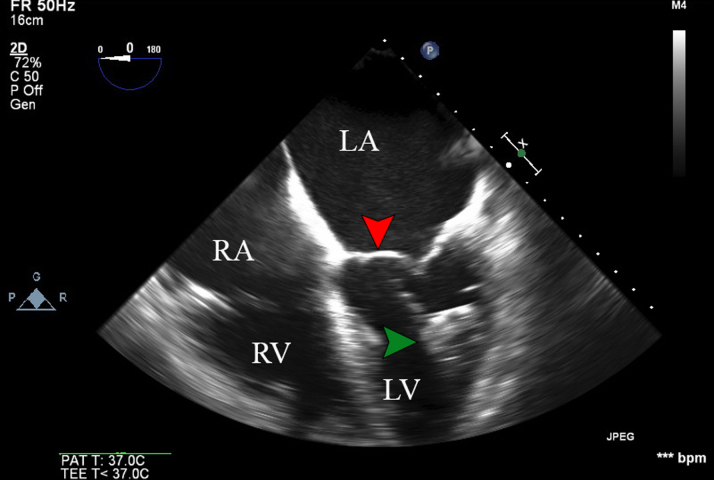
TEE Midesophageal 4-Chamber View Showing an Embolized Watchman Device (Green Arrowhead) Lodged in the Left Ventricle The red arrowhead indicates the mitral valve. LA = left atrium; LV = left ventricle; RA = right atrium; RV = right ventricle; TEE = transesophageal echocardiography.
